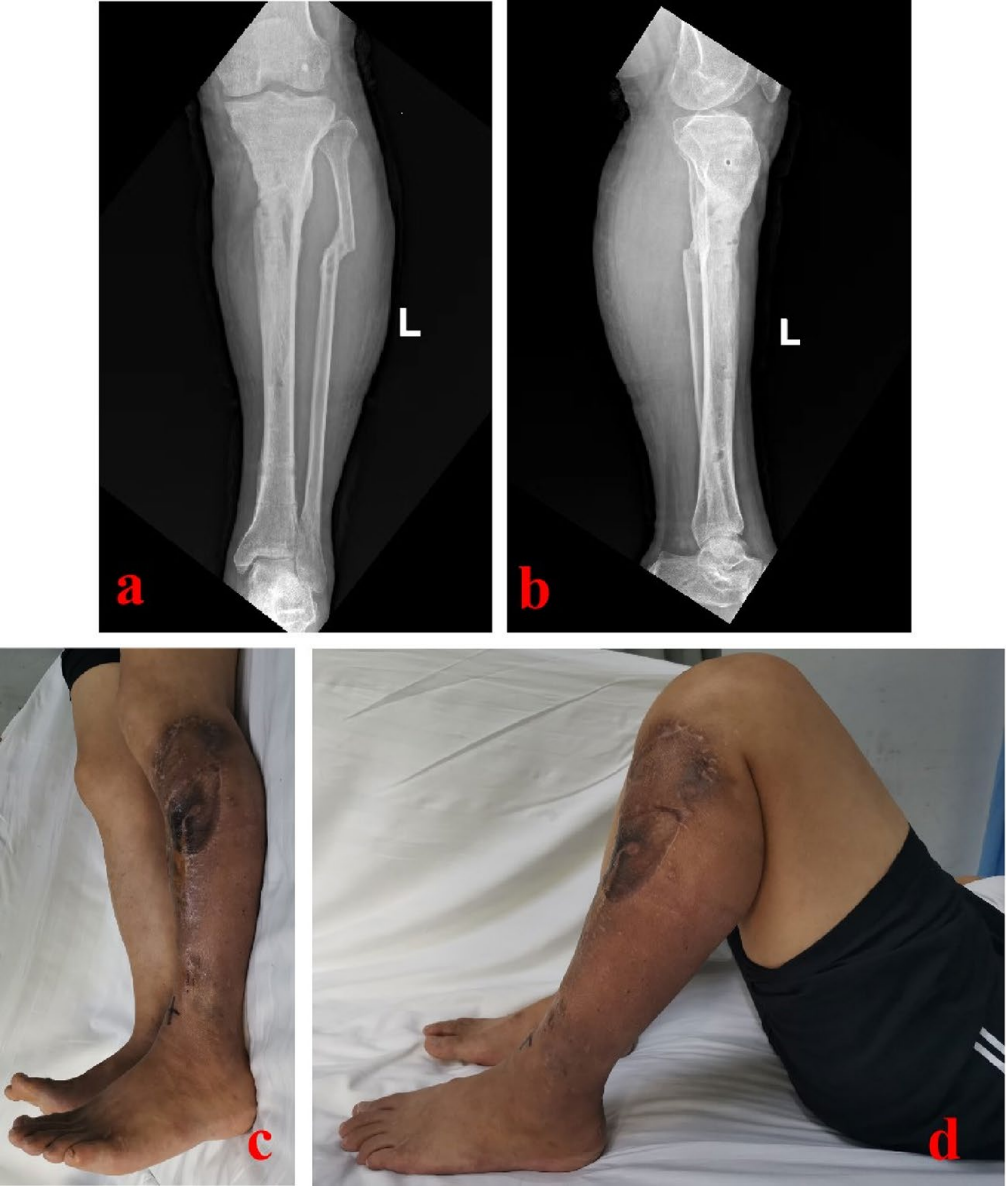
The patient was successfully treated by the modified bone transport technique. (a) and (b) X-ray films after removal of the annular frame; (c) and (d) Appearance of the injured limb after removal of the annular frame.
